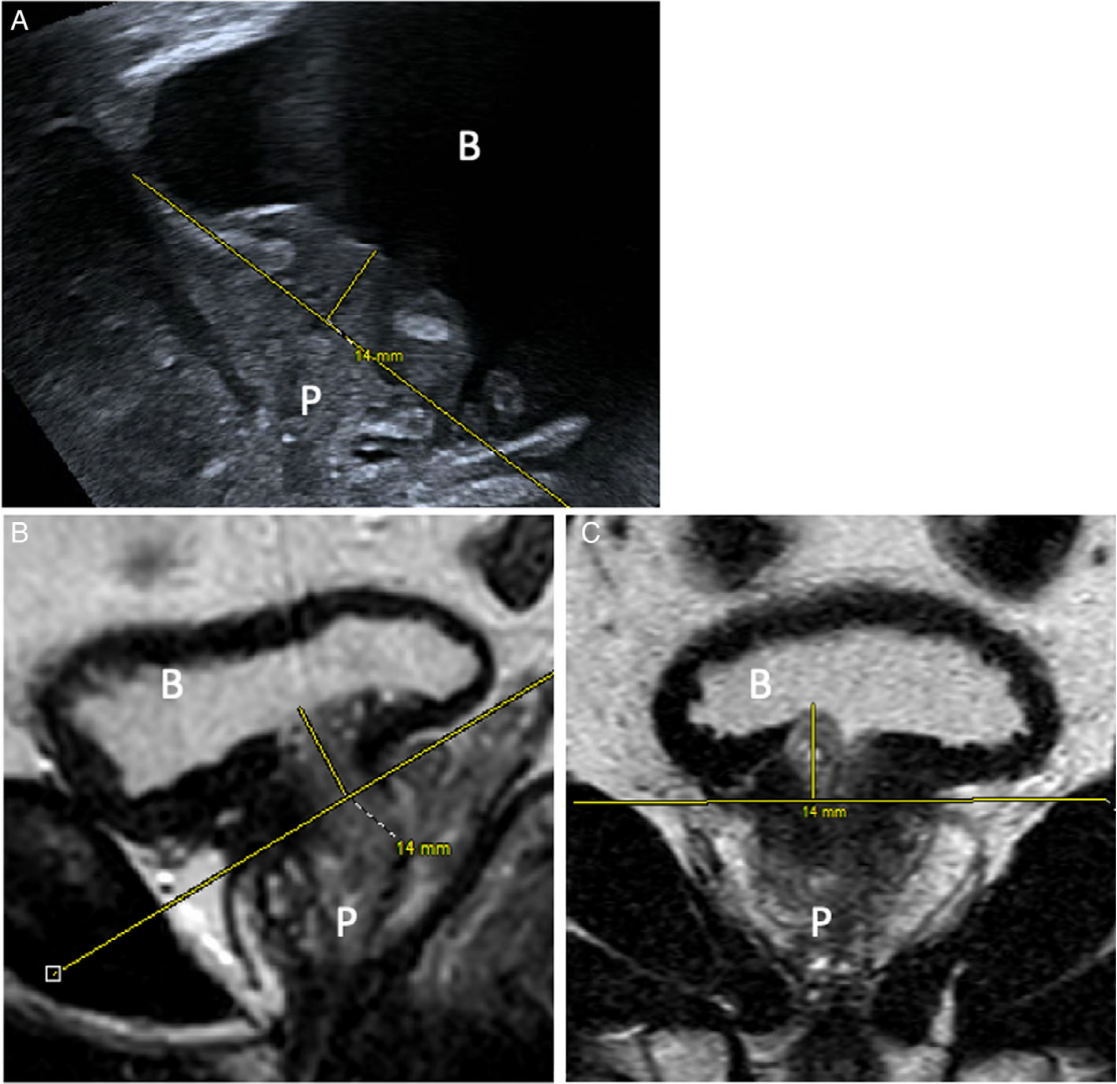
Pre‐operative TPUS image in the longitudinal plane (A) and mp‐MR images in sagittal (B) and coronal plane (C). The images show the intravesical prostate protrusion assessed by the two techniques. The letter “B” indicates the bladder and the “P” the prostate. In figure part A, the right‐hand side of the image is anterior and the left posterior to the patient.
